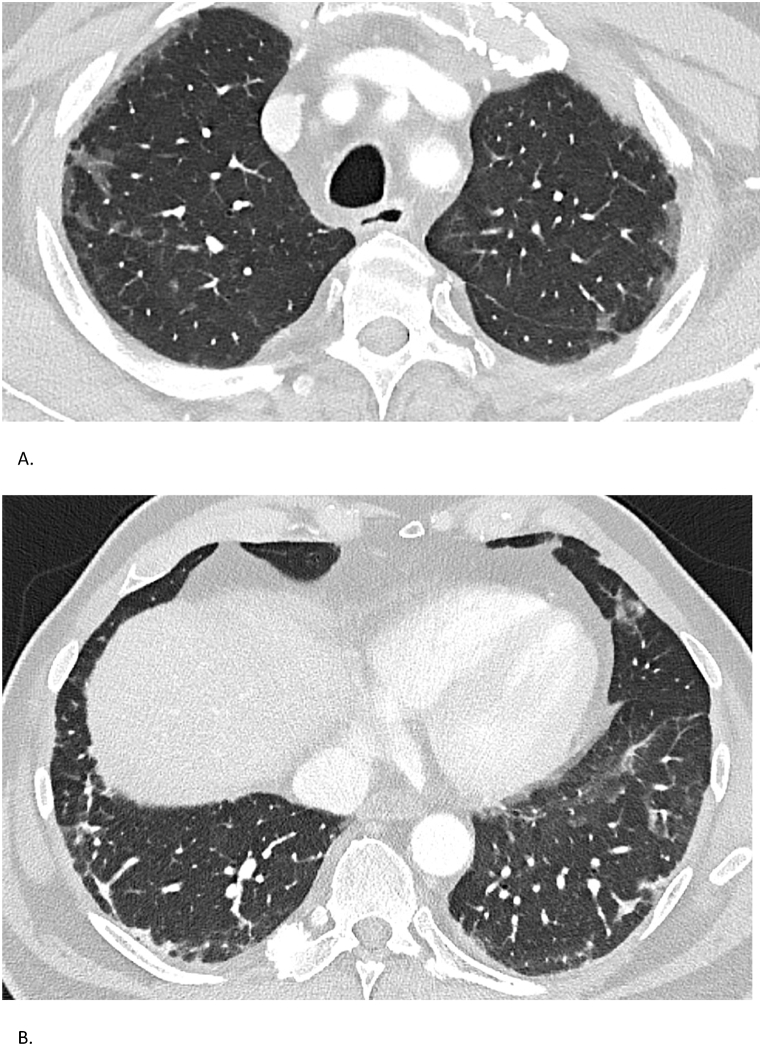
Case 3 – Chest CT findings Serial chest CTs of a 69-year-old man with *anti*-MDA5-positive ILD. (A) CT on May 3, 2023, displays subpleural fibrosis and diffuse ground-glass opacities. (B) CT on the same date in a different plane confirms extensive fibrotic changes without honeycombing.
